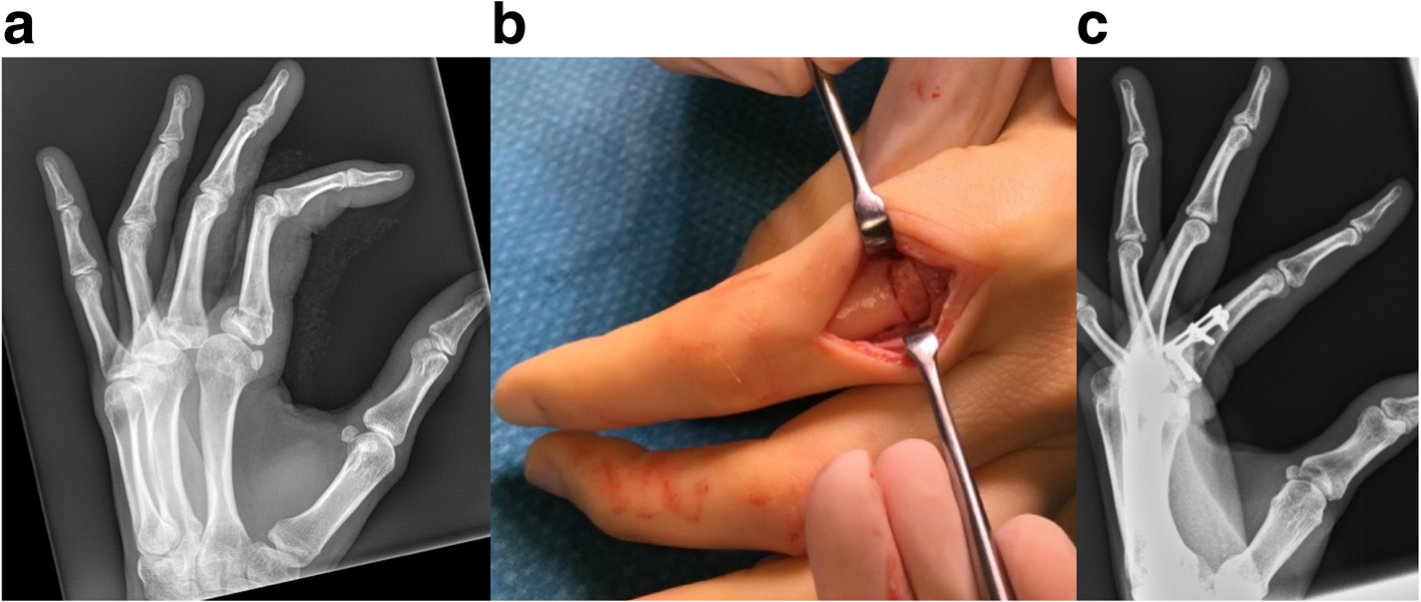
Pat. #11: a Pre-operative radiographic study. Patient suffered from an extension deformity following a fracture of the proximal phalanx of the index finger. b After multiple-drill hole osteotomy an iliac crest autograft was inserted followed by plate osteosynthesis. c Radiographic study 1 month post-operative
